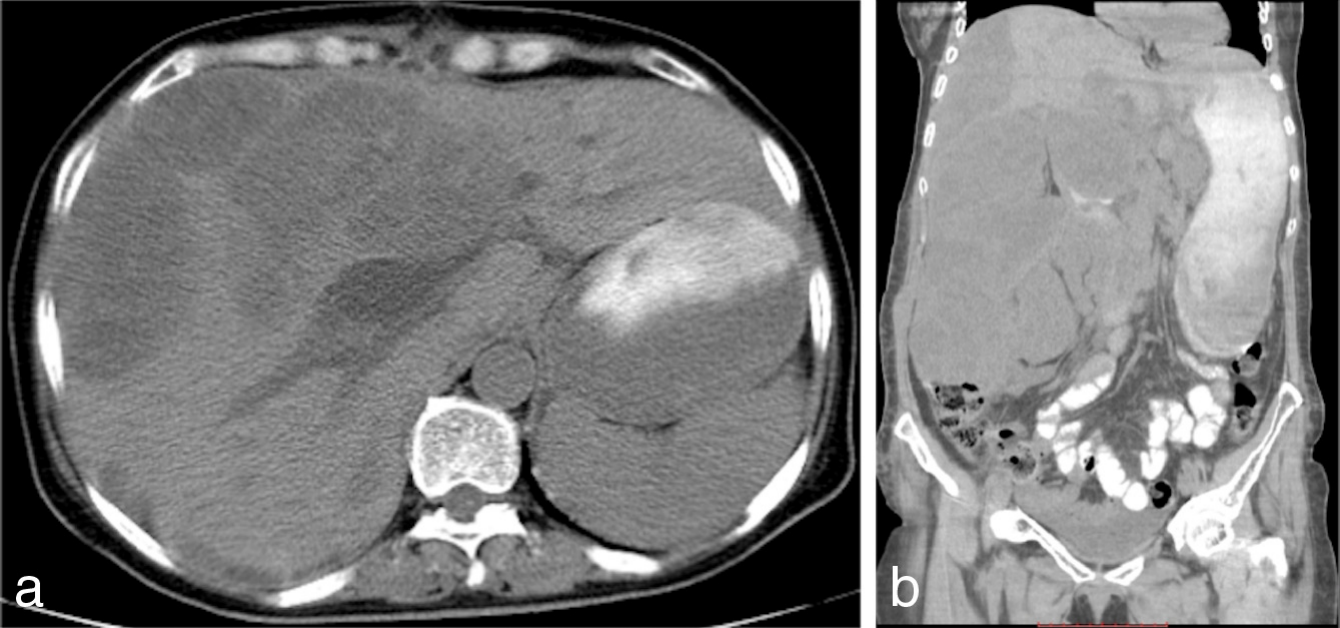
Axial (a) and coronal reformatted (b) images of non-enhanced multidetector CT show the presence of multiple hypodense lesions in the left hepatic lobe, as well as multiple nodular, solid hypodense intra-abdominal lesions compatible with recurrence of the resected carcinosarcoma of the gallbladder.
